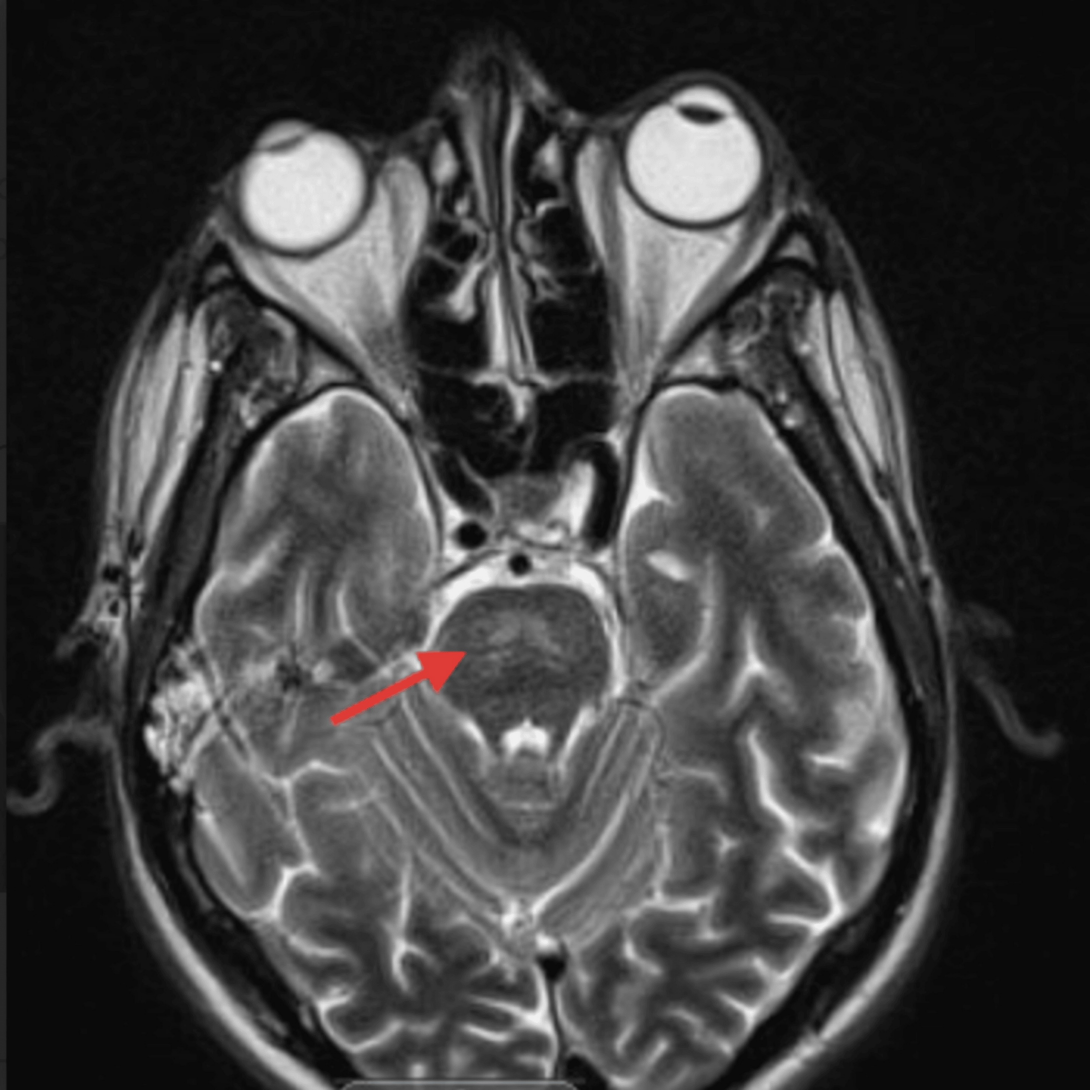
Brain MRI - image 1 The image shows T2-weighted FLAIR hyperintense lesions within the central pons, resembling an incomplete trident sign (arrow) MRI: magnetic resonance imaging: FLAIR: fluid-attenuated inversion recovery
